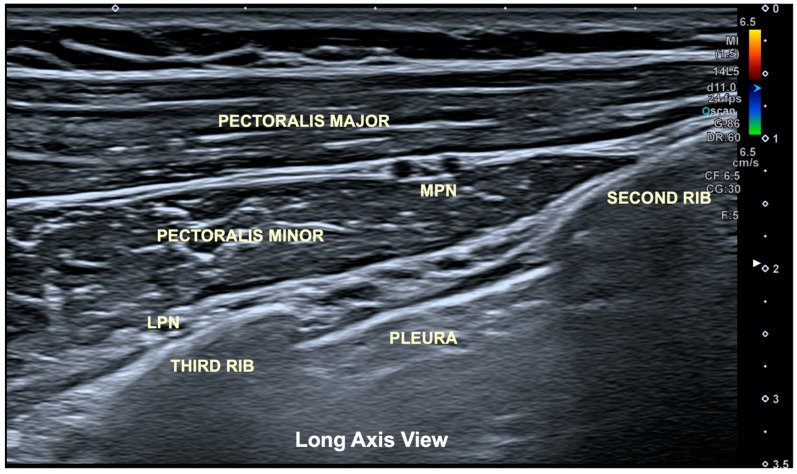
Longitudinal axis ultrasound image showing the “bat sign” (between the second and third rib). Using this anatomical ultrasound view as a reference, the muscle bellies of both pectoralis major and pectoralis minor muscles and the parietal pleura can be properly visualized. MPN: medial pectoral nerve; LPN: lateral pectoral nerve.
